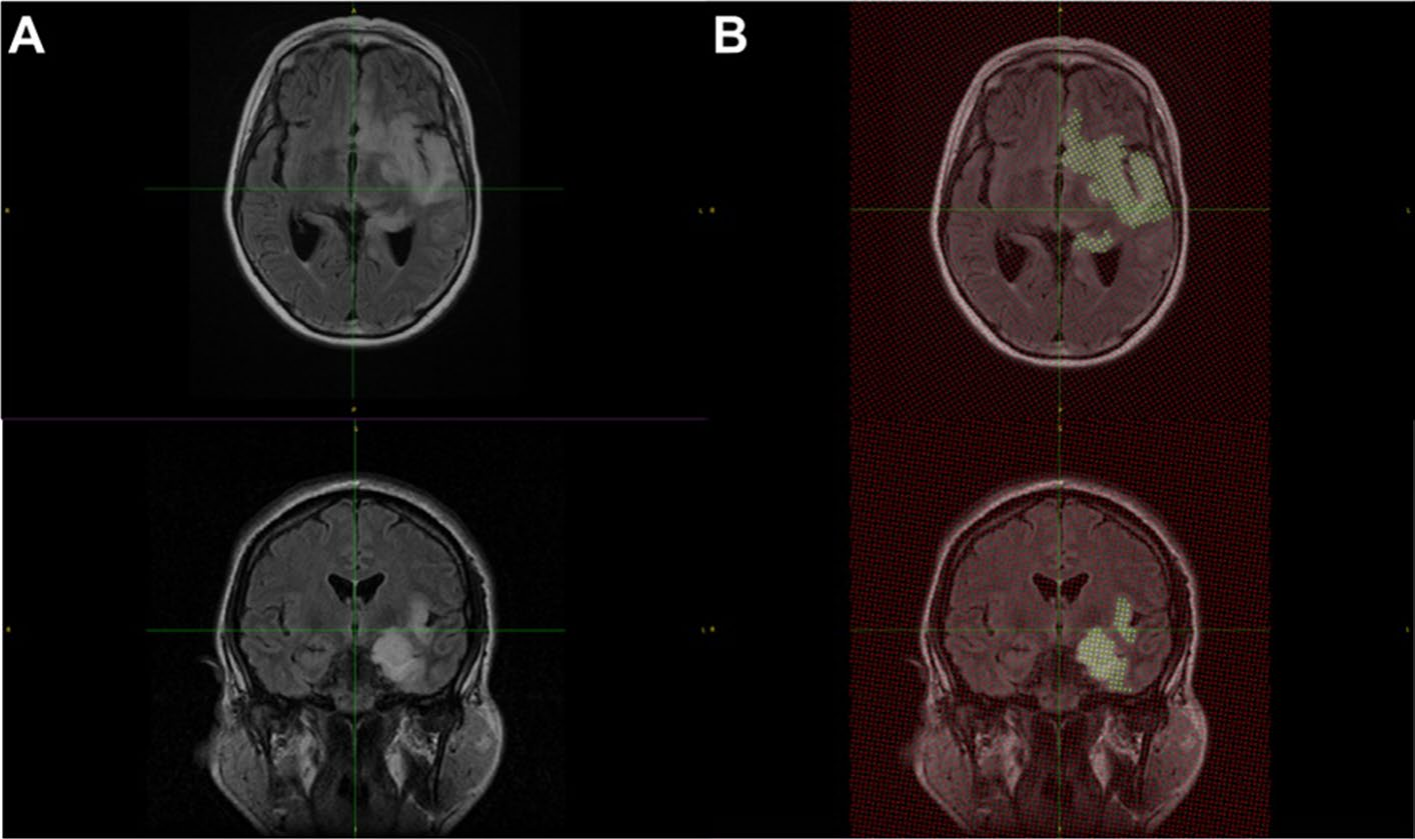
A MRI coronal and axial slices showing areas of cortical oedema in patients with acute encephalitis syndrome, B The same MRI slices analysed on EasyMeasure using point counting with stereology; yellow dots signify areas of oedema and are stereologically analysed to create a volume of oedema
